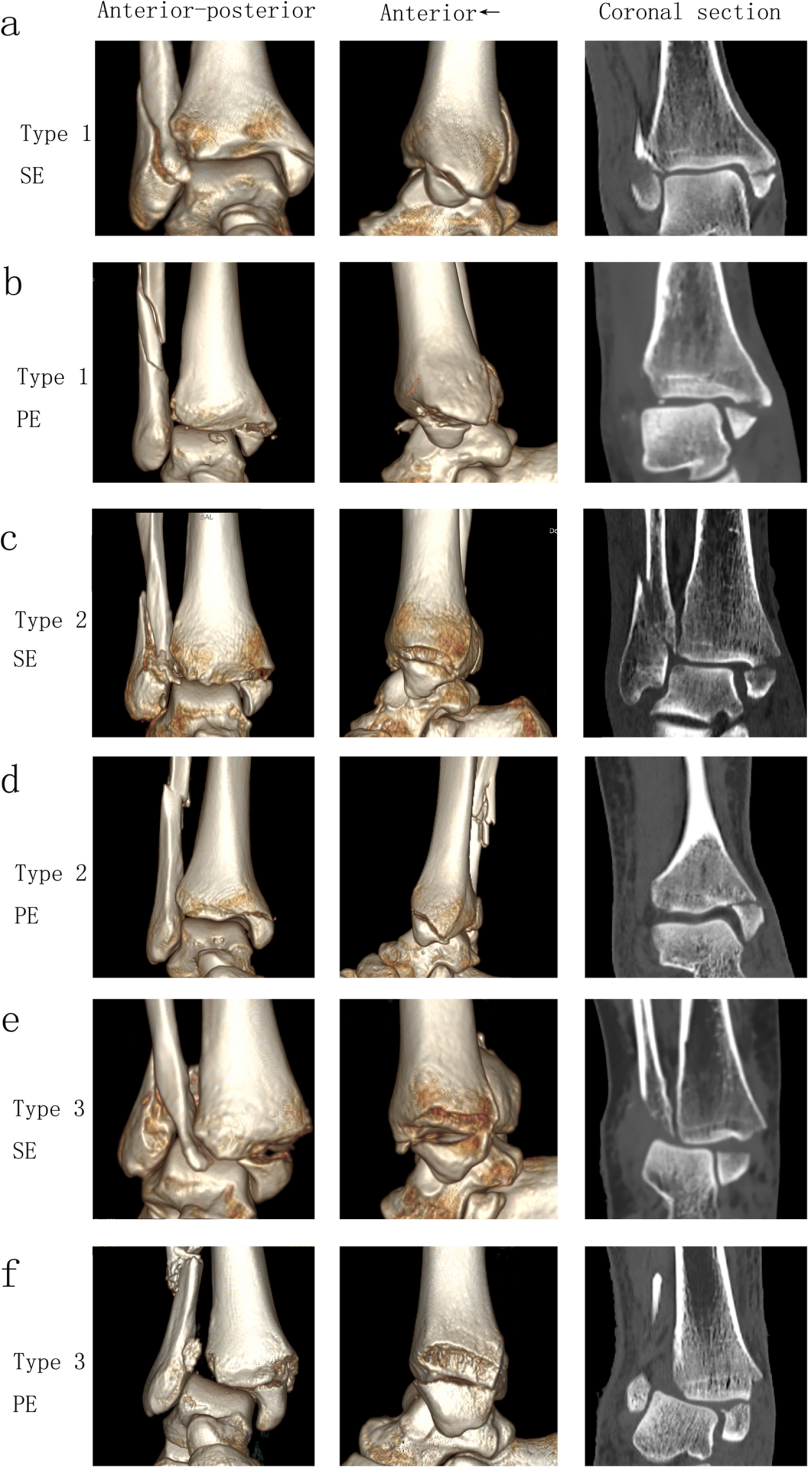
Representative 3-D reconstruction CT images of type 1/2/3 in cases of Lauge-Hansen supination-external rotation (SE) compared with pronation-external rotation (PE). a Type 1 of SE. b Type 1 of PE. c Type 2 of SE. d Type 2 of PE. e Type 3 of SE. f Type 3 of PE
